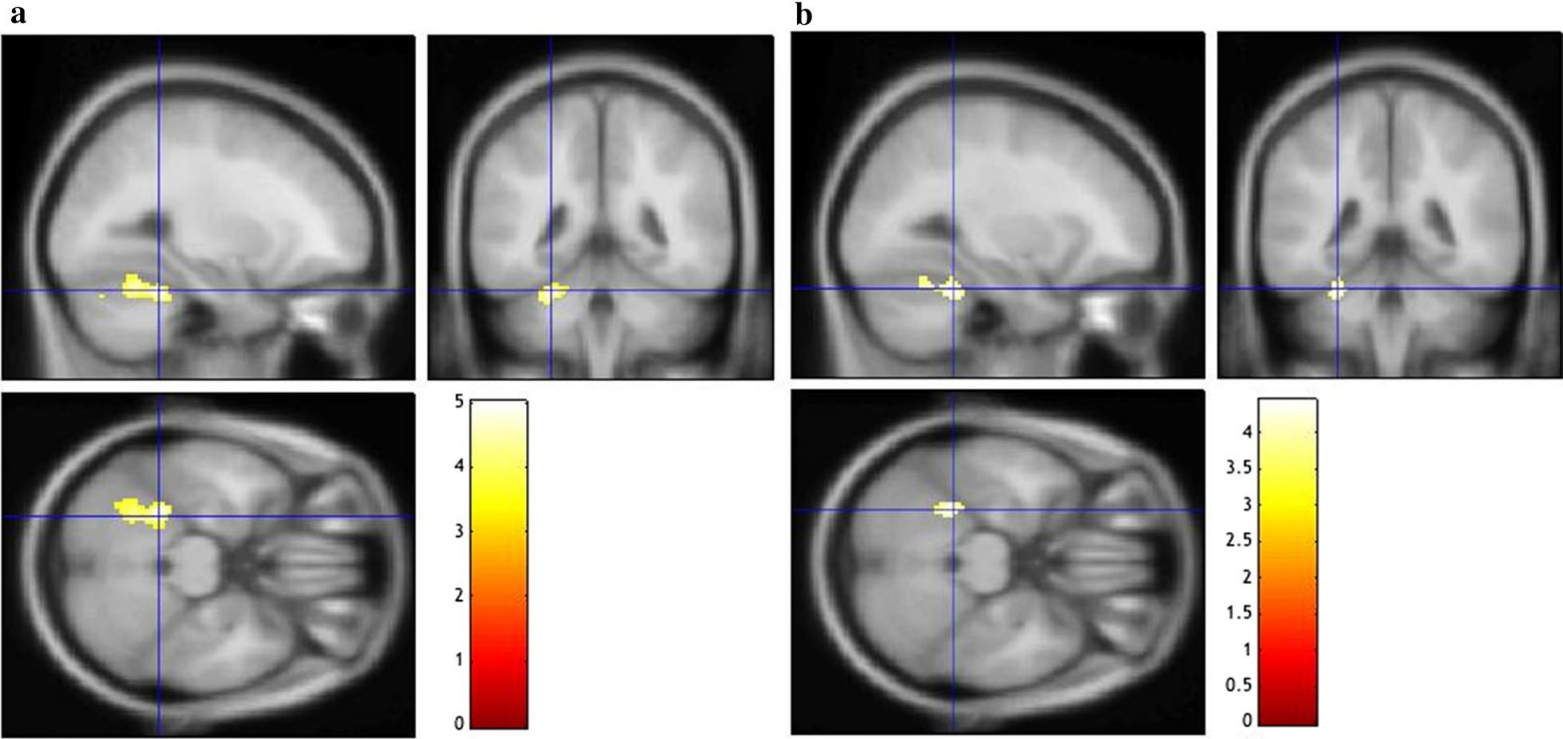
a Regions in which cerebral metabolism were significantly and inversely associated with log-transformed ambient light. A whole brain analysis revealed there was a significant reduction of FDG activity with log-transformed ambient light in the left cerebellum, which was overlaid on a T1 weighted MRI template of SPM8. Blue rectangular coordinates indicated a peak level the left cerebellum (MNI coordinates: x, y, z = − 26, − 44, − 26). A color bar shows T value. b Regions in which cerebral metabolism was significantly and inversely associated with log-transformed ambient light after adjustment for age, gender and serum melatonin levels. After adjustment for age, gender and serum melatonin levels, there was a significant reduction of FDG activity with log-transformed ambient light in the left cerebellum, which was overlaid on a T1 weighted MRI template of SPM8. Blue rectangular coordinates indicated a peak level the left cerebellum (MNI coordinates: x, y, z = − 28, − 42, − 26). A color bar shows T value
